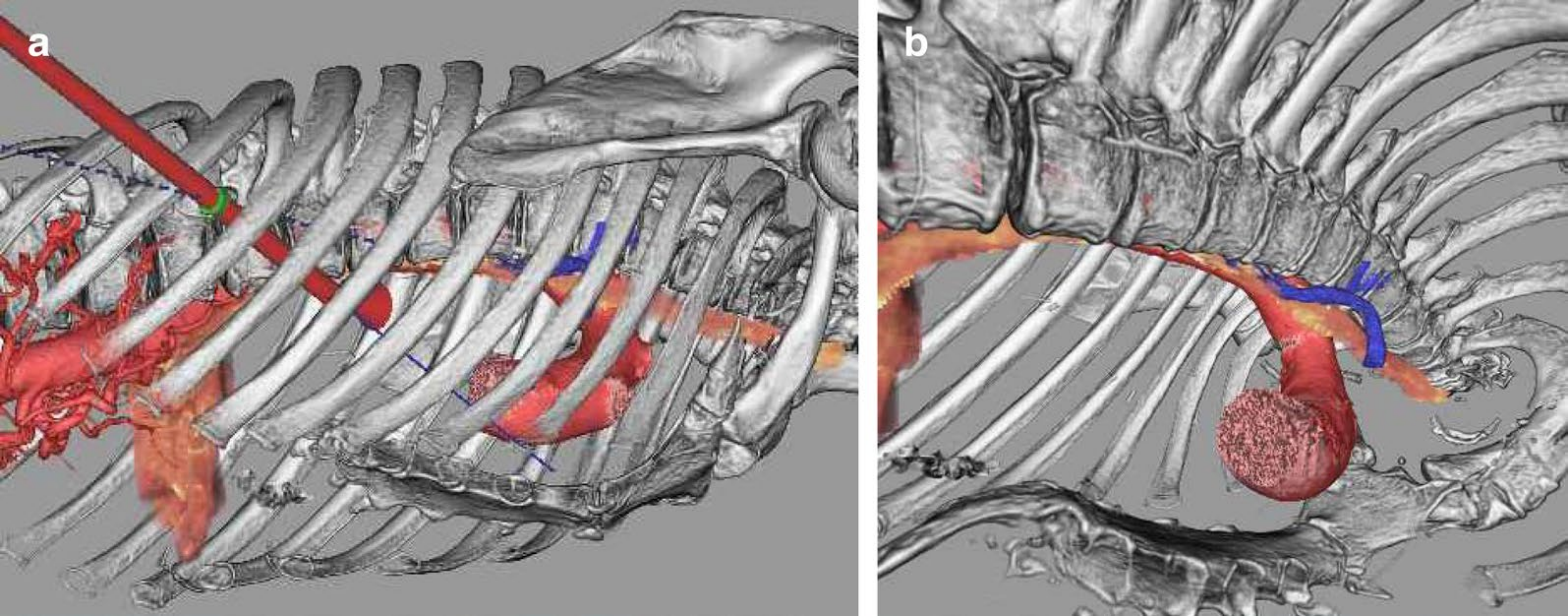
a 3D-CT image created by contrast-enhanced CT images in the supine position. b 3D-CT imaging revealed difficulty in ensuring the surgical field
